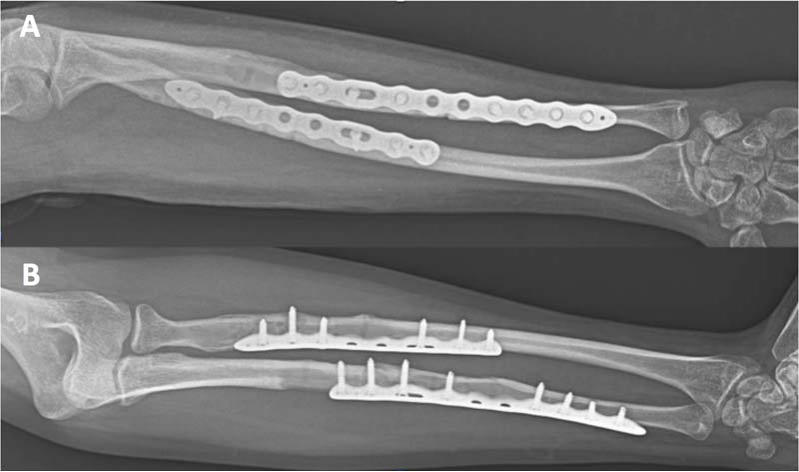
Internal fixation of both NPPIFs with a longer plate that bridges the original fracture zone and the new NPPIF. Final follow-up at 36 months after surgery, anterior–posterior X-ray.
